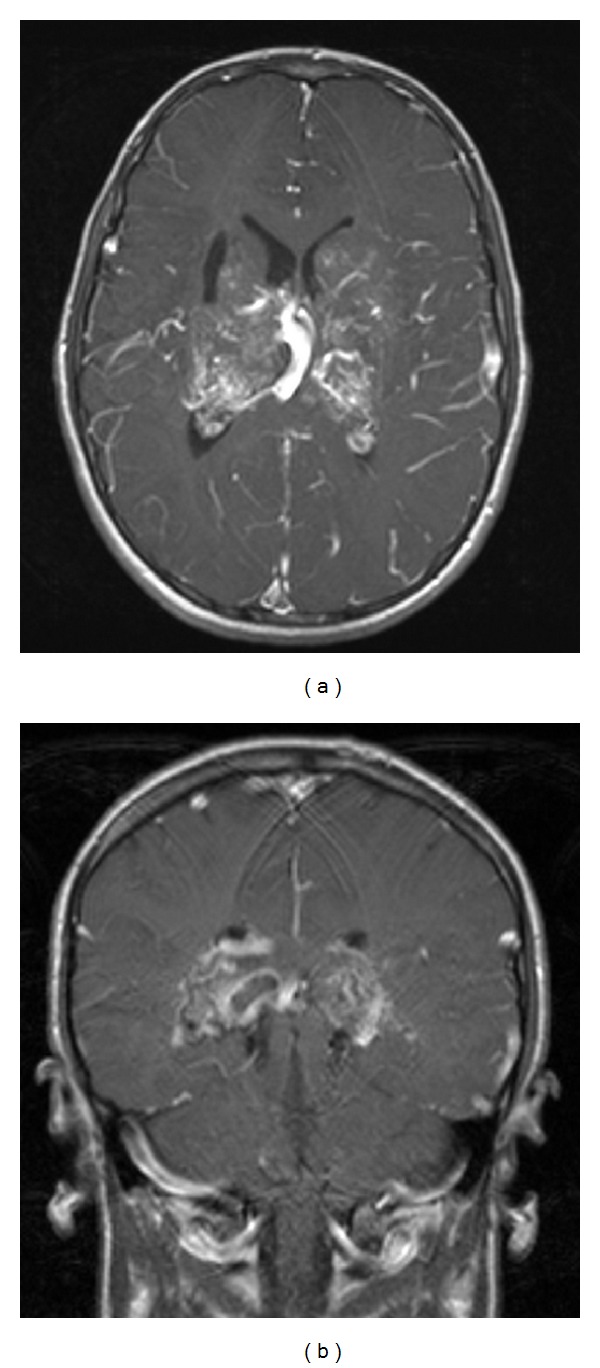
MRI at initial presentation. (a) T1-weighted, axial, gadolinium-enhanced image at the level of the foramen of Monro. (b) T1-weighted, coronal, gadolinium-enhanced image posterior to the third ventricle.
